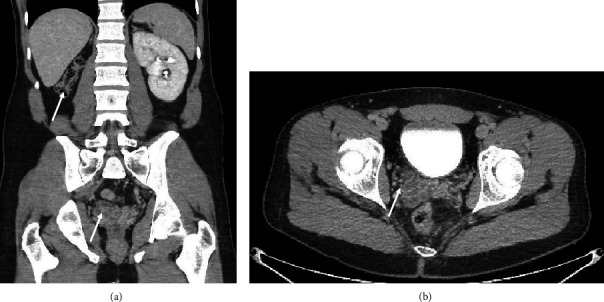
Abdominopelvic computerized tomography (CT). (a, b) Coronal and axial images reveal atrophic right kidney with cystic right seminal vesicle.
